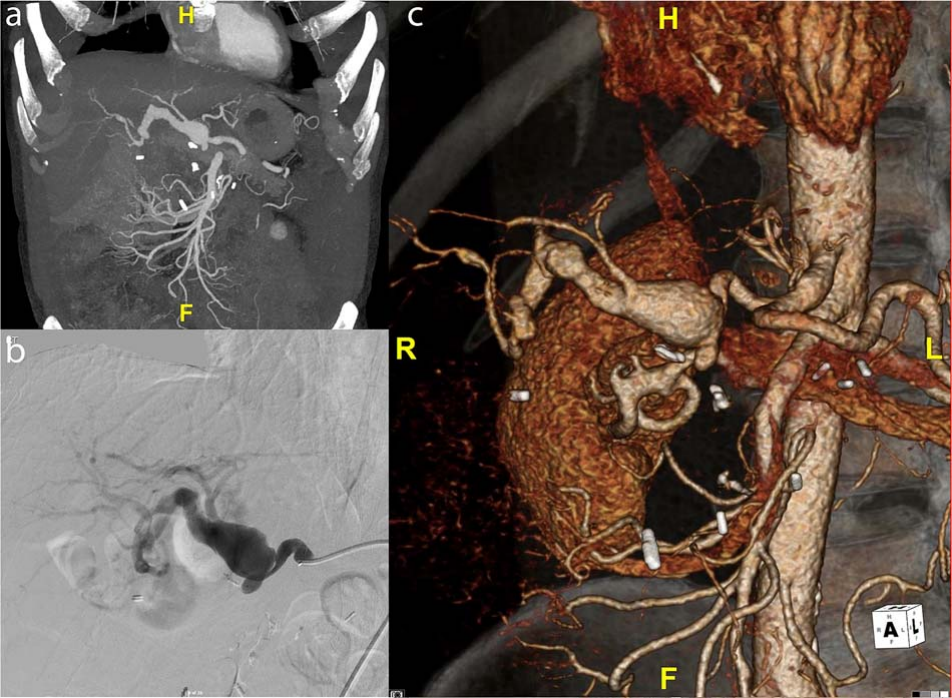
(a) Preoperative CT scan of hepatic artery aneurysm postremote pancreaticoduodenectomy. (b) Catheter-based angiogram of hepatic aneurysm. (c) 3-D reconstruction of hepatic artery aneurysm.
